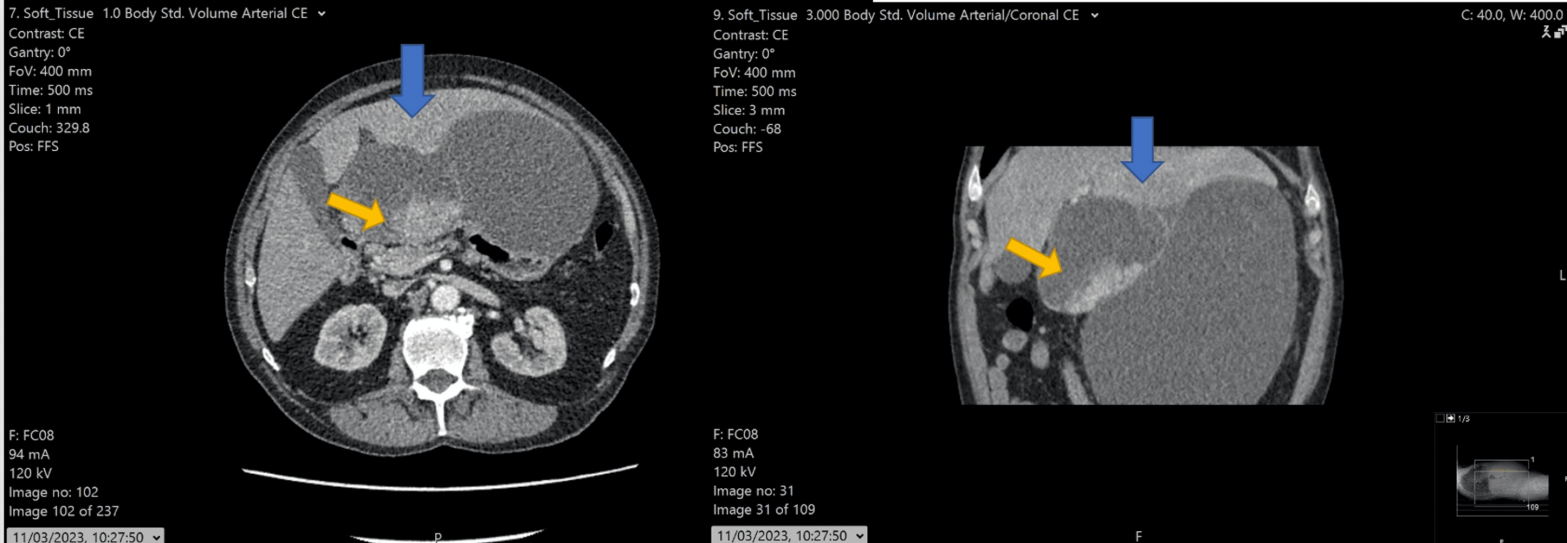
Hepatic angiosarcoma (HA) mimicking a complex liver cyst Axial and coronal imaging of CECT showing a large cystic lesion arising from the left lateral segment of the liver expanding between the liver, pancreas, and stomach (blue arrow). Some soft tissue components are evident (yellow arrow); however, in the context of the bi-lobar cystic lesion, this was interpreted initially as cyst wall complexity. HA: Hepatic angiosarcoma: CECT: Contrast-enhanced computed tomography
